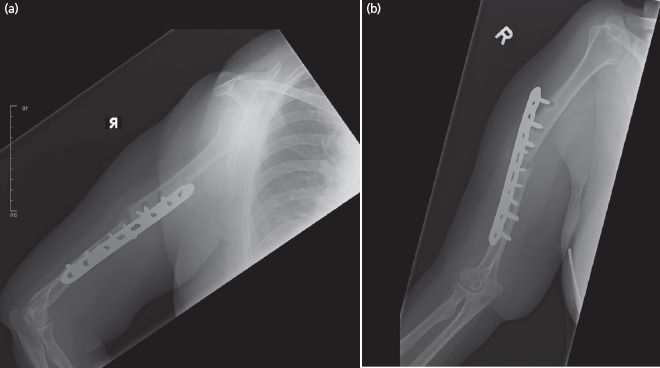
Radiographs taken at nine months post-primary surgery. En masse proximal screw pull-out of previous locking plate fixation (AP and lateral view). The most proximal screw was confirmed to be intracortical. The second and third screws were unicortical.
